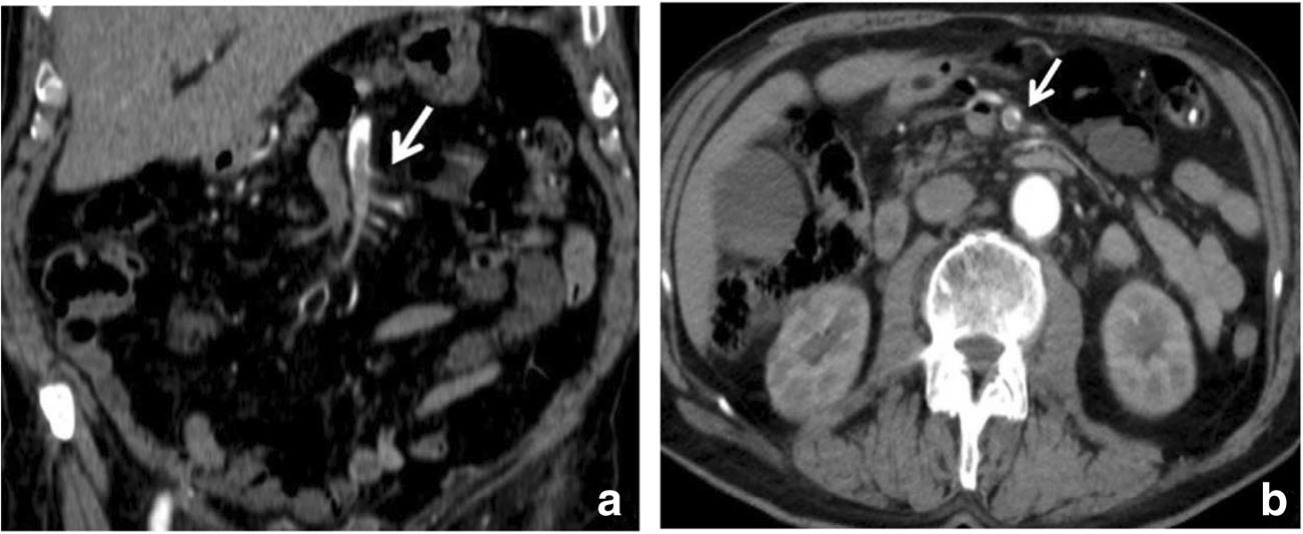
Arterial occlusion. Contrast-enhanced CT image of the abdomen in a 54-year-old female with superior mesenteric arterial thrombosis. a, b: Contrast-enhanced axial CT scan demonstrates a thrombus in the superior mesenteric artery in coronal (a) and axial plane (b) (white arrow)
